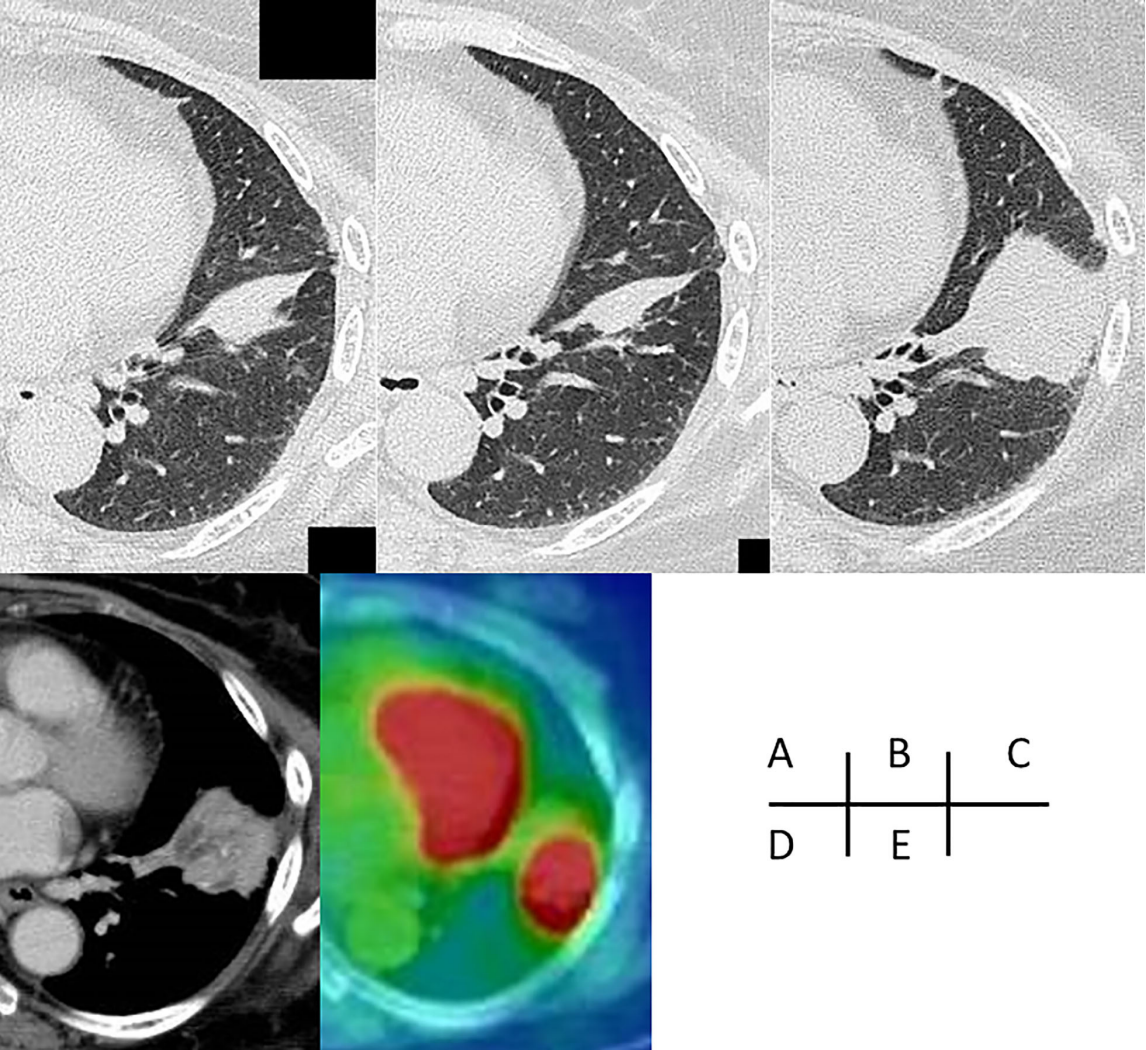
Temporal changes to the mass on chest computed tomography (CT) (A, 3 years previously; B, 1 year previously; C, preoperative image). Preoperative CT shows a mass, 45 × 37 × 32 mm, and contrast effects are seen on the margins of the mass (C, D). The mass shows a maximum standardized uptake value of 8.91 on 18F‐fluorodeoxyglucose‐positron emission tomography (E)
